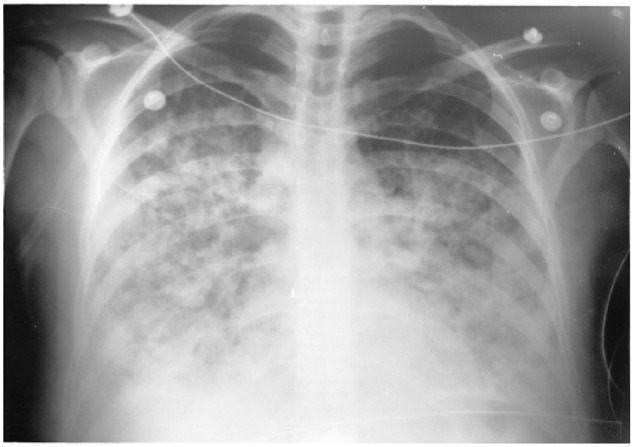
3rd Chest radiograph, done in ICU.
